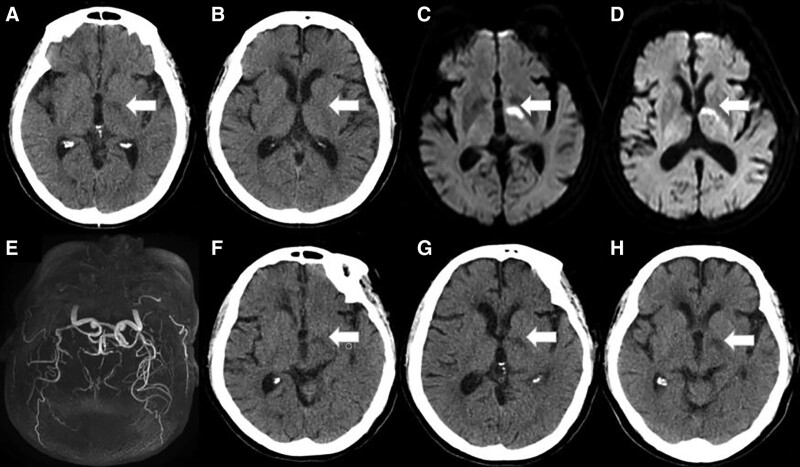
A and B, there is no hemorrhage. A low-density image of the anterior reticular nucleus of the left thalamus can be seen at the level of the third ventricle. C, D shows the limited diffusion of the left thalamic reticular nucleus. E there is no stenosis or occlusion of large blood vessels in the anterior intracranial circulation. F and G images show low-density visualization of the left thalamic reticular nucleus area after thrombolysis, which is slightly larger than before. Only a few low-density areas are left in one CT slice in the H image. CT = computed tomography.
